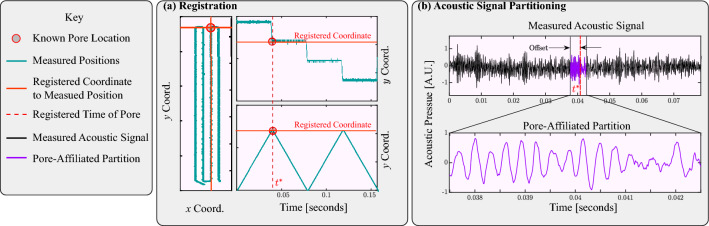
The spatial-temporal registration scheme involves two main steps. First, the pore location(s) identified in radiography images are registered to the corresponding laser-measured locations on an $$x-y$$ coordinate grid (a). Second, the time(s) at which the measured *x* and *y* coordinates align with a registered pore are denoted as $$t^*$$, representing the registration time(s) (b). The acoustic signal is then partitioned into a pore-affiliated segment based on the registered pore time (*t*) and a random offset. This segmentation allows for the isolation and analysis of the acoustic data associated with the registered pore(s).
